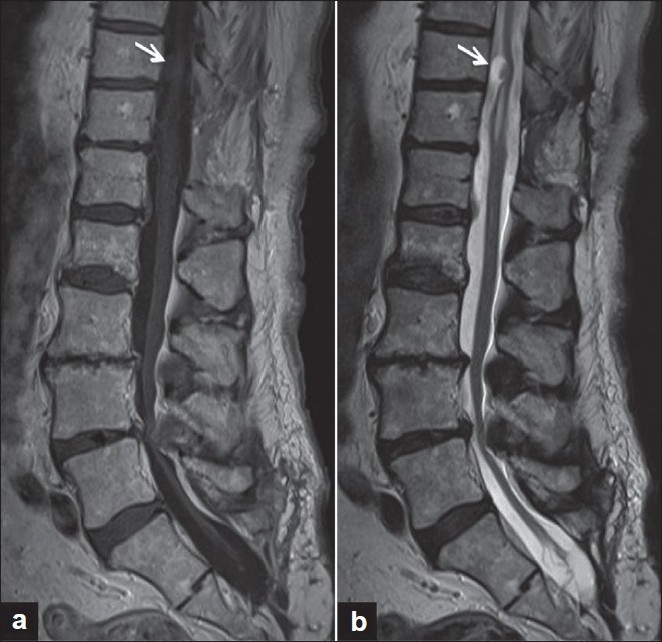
MRI of thoracolumbar junction with intradural/ extramedullary neurenteric cyst at T11. (a) Midsagittal MRI demonstrates isointense lesion (arrow) on T1-weighted and (b) hyperintense signal (arrow) on T2-weighted
